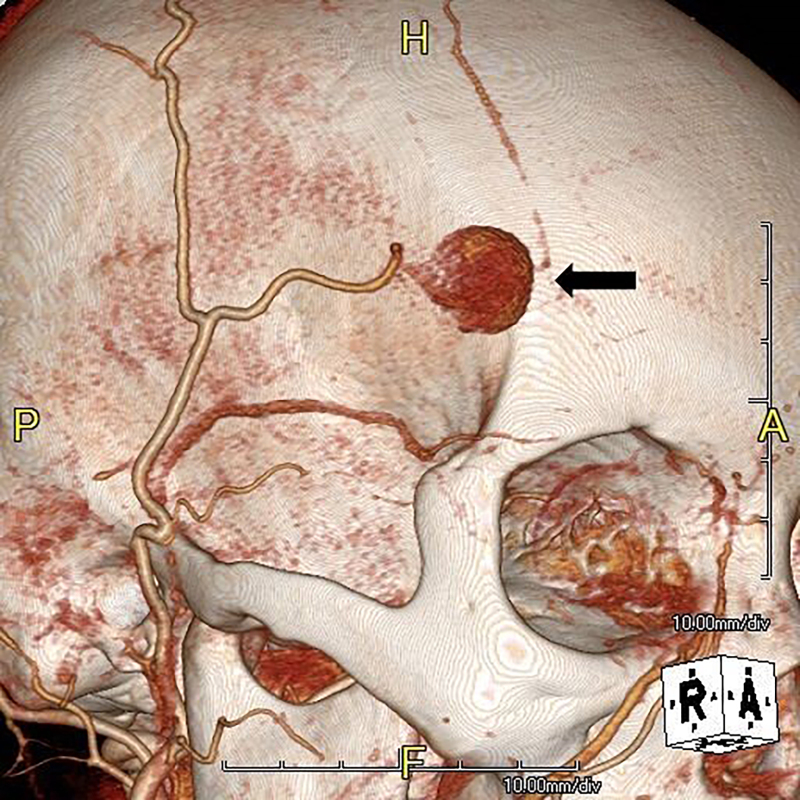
Three-dimensional computed tomography angiogram demonstrating a large superficial temporal arterial pseudoaneurysm (arrow).
